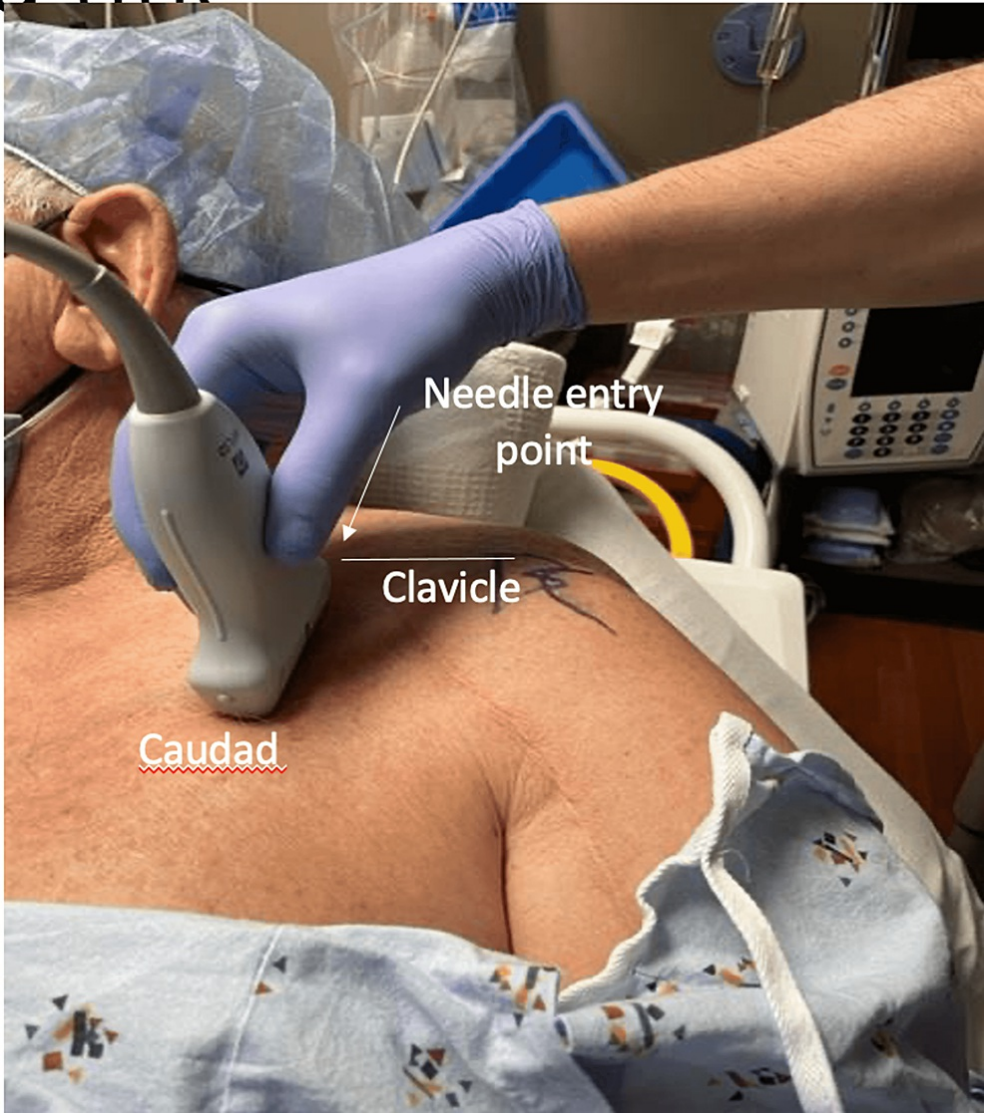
Patient positioned supine with the head turned away from the block side. The linear ultrasound probe placed under the clavicle in the deltopectoral groove for the in-plane needle infraclavicular technique.
